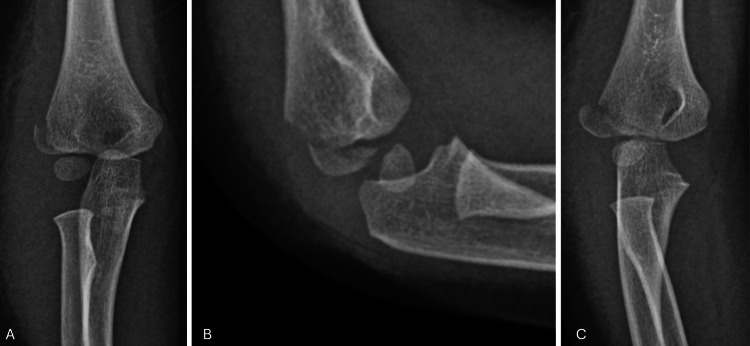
(A) Anteroposterior (AP), (B) lateral, and (C) oblique views of right elbow radiographs. AP = anteroposterior.
